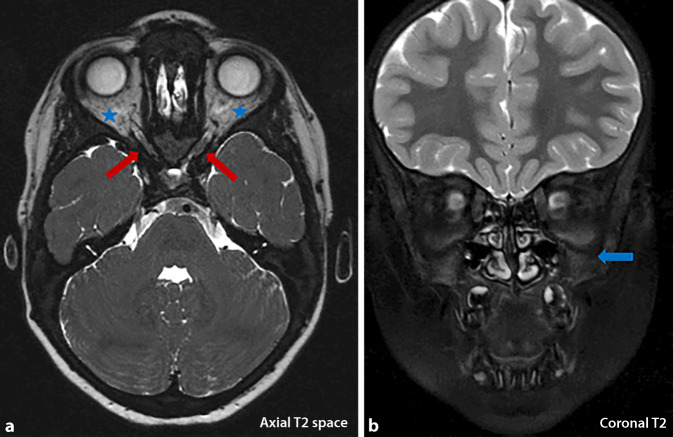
Brain MRI showing typical findings in patients with osteopetrosis. a On the right emphasized, pronounced narrowing of the optic canal with accompanying atrophy of the optic nerve on both sides (*blue stars*). The sheath of the optic nerve can no longer be delimited within the channel (*red arrows*). b Complete lack of pneumatization of the paranasal sinuses (*blue arrow*)
